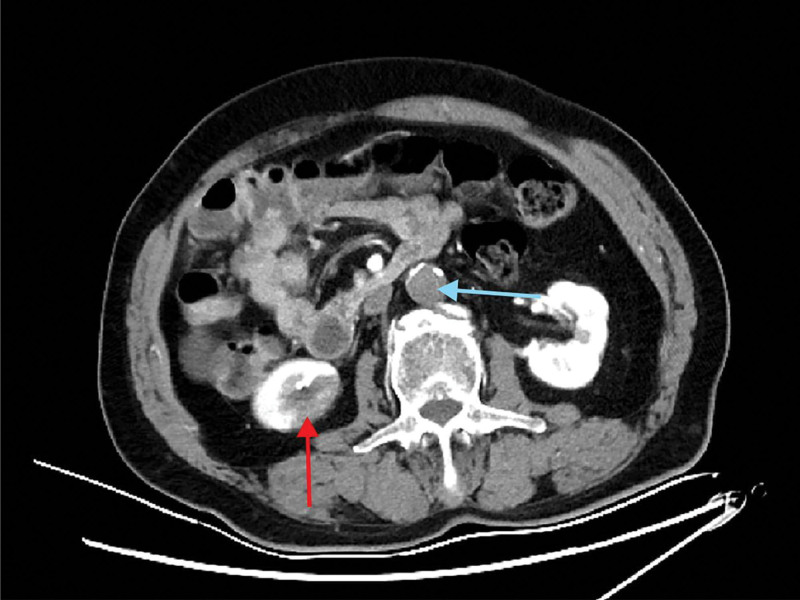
Computed tomography angiography of the chest and abdomen reveals thrombus formation and occlusion within the abdominal aorta (blue arrow); localized infarction of the lower pole of the right kidney (red arrow).
